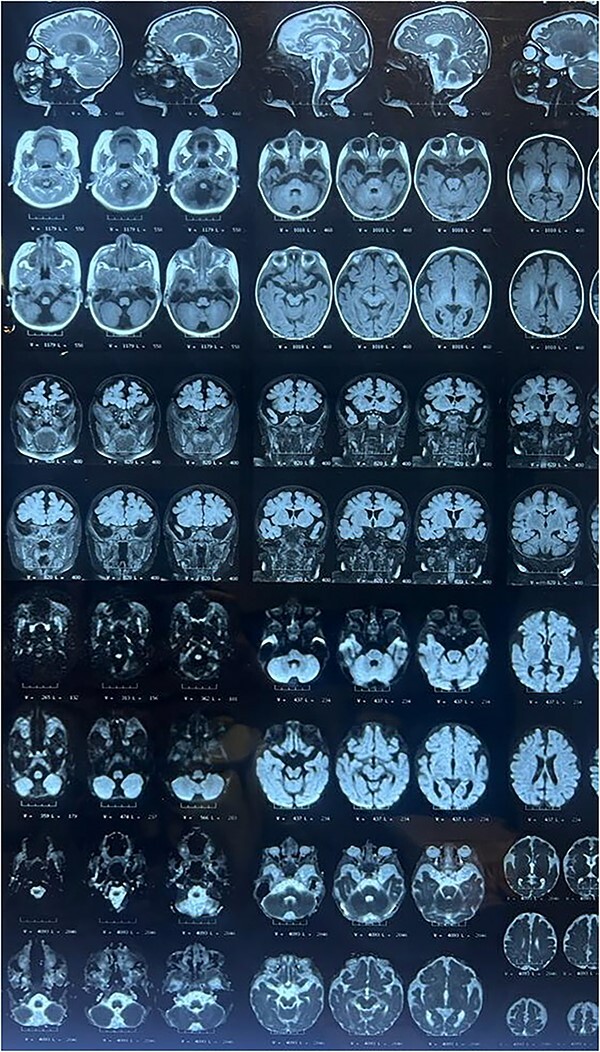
Brain MRI: diffuse white matter in the periventricular, subarachnoid and juxtacortical areas, as well as brain stem, putamen, cerebellum and bilateral thalami involvement.
